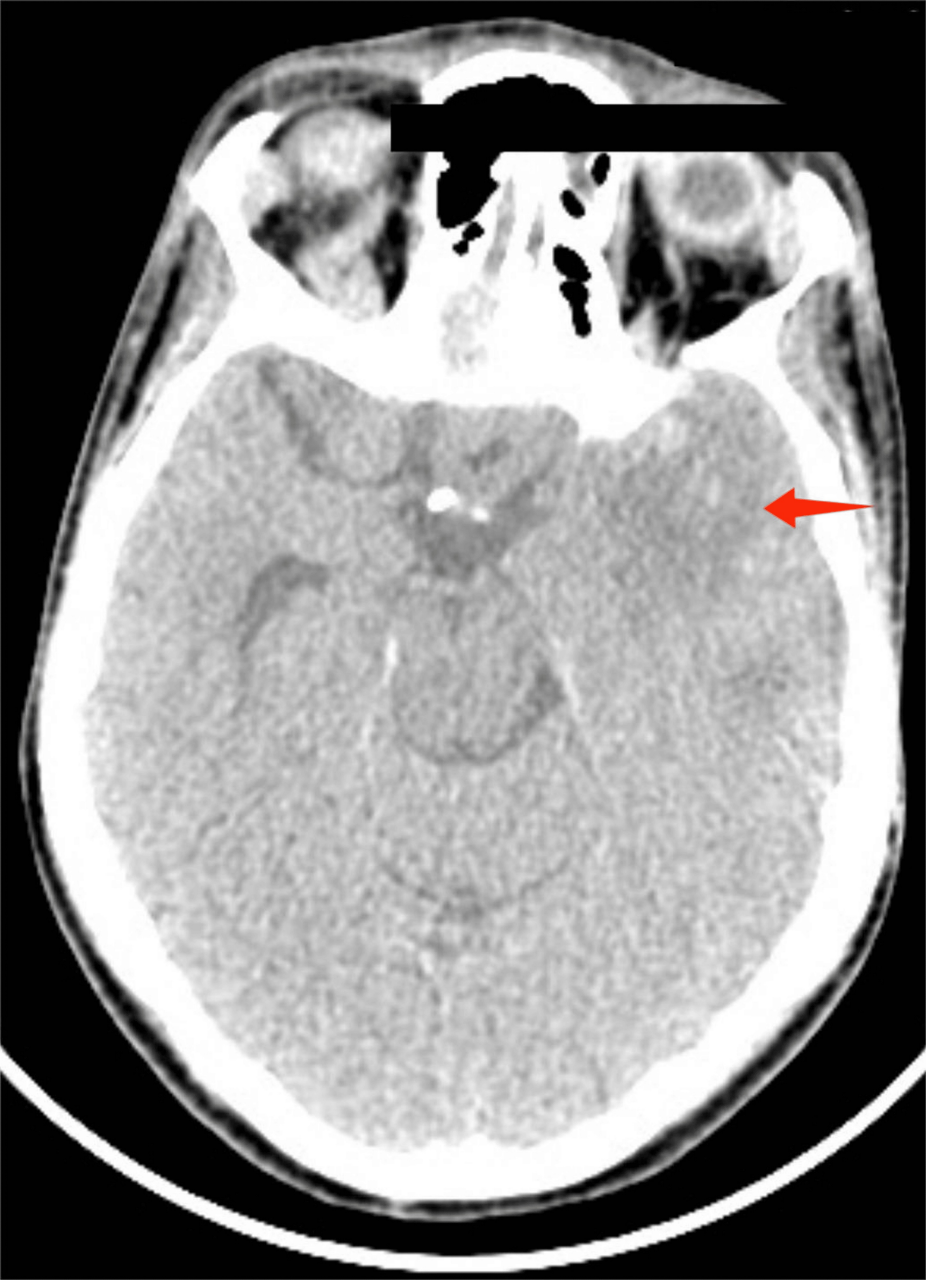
CT brain without contrast-axial view; the red arrow shows the dense left middle cerebral artery thrombus
